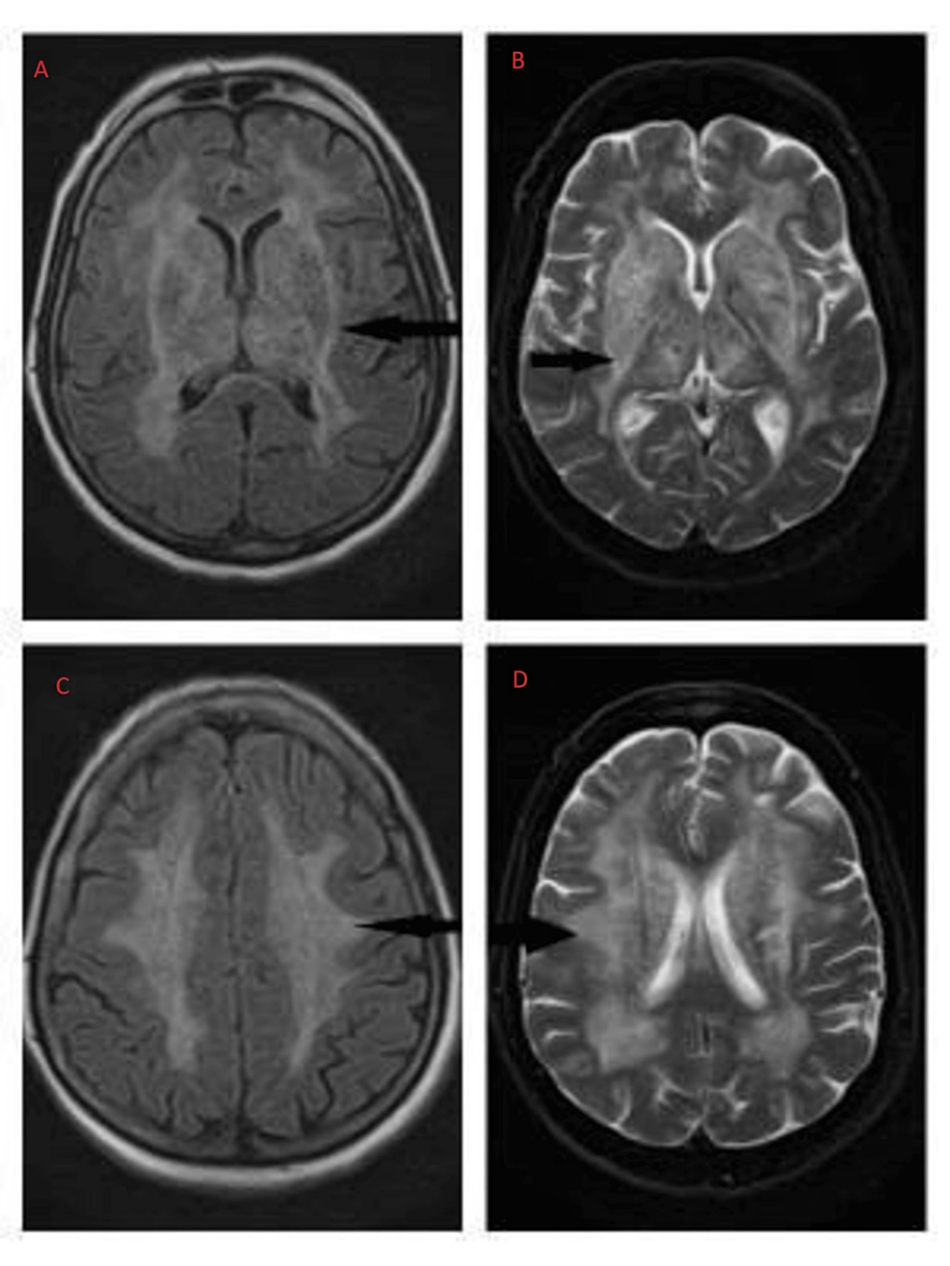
Brain MRI on follow-up: T2WI and FLAIR images in axial view showing minimal interval reduction in bilateral symmetrical hyperintensities of BG and deep and subcortical white matter. MRI performed on the fourth month of follow-up. (A, B) Symmetrical T2/FLAIR hyperintense signals in BG, thalami, and internal and external capsules. (C, D) Interval static extensive T2/FLAIR hyperintensities in bilateral periventricular, deep, and subcortical white matter. BG: basal ganglia.
